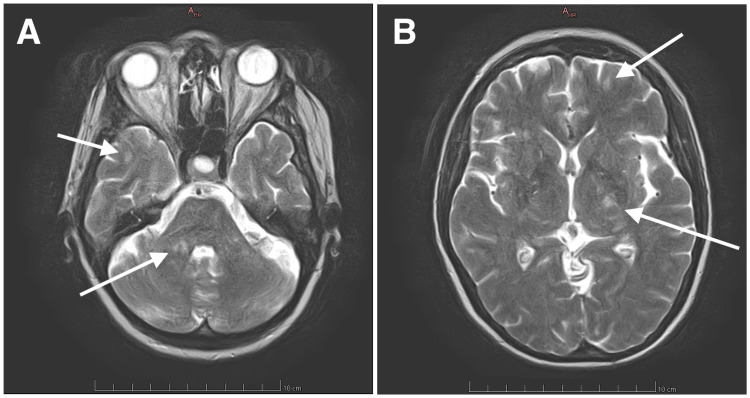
Initial cerebral MRI exhibiting multiple T2-weighted hyperintense white matter lesions (arrows) on the middle right cerebellar peduncle and right temporal lobe (Panel A) and posterior limb of the left internal capsule and left frontal lobe (Panel B).
